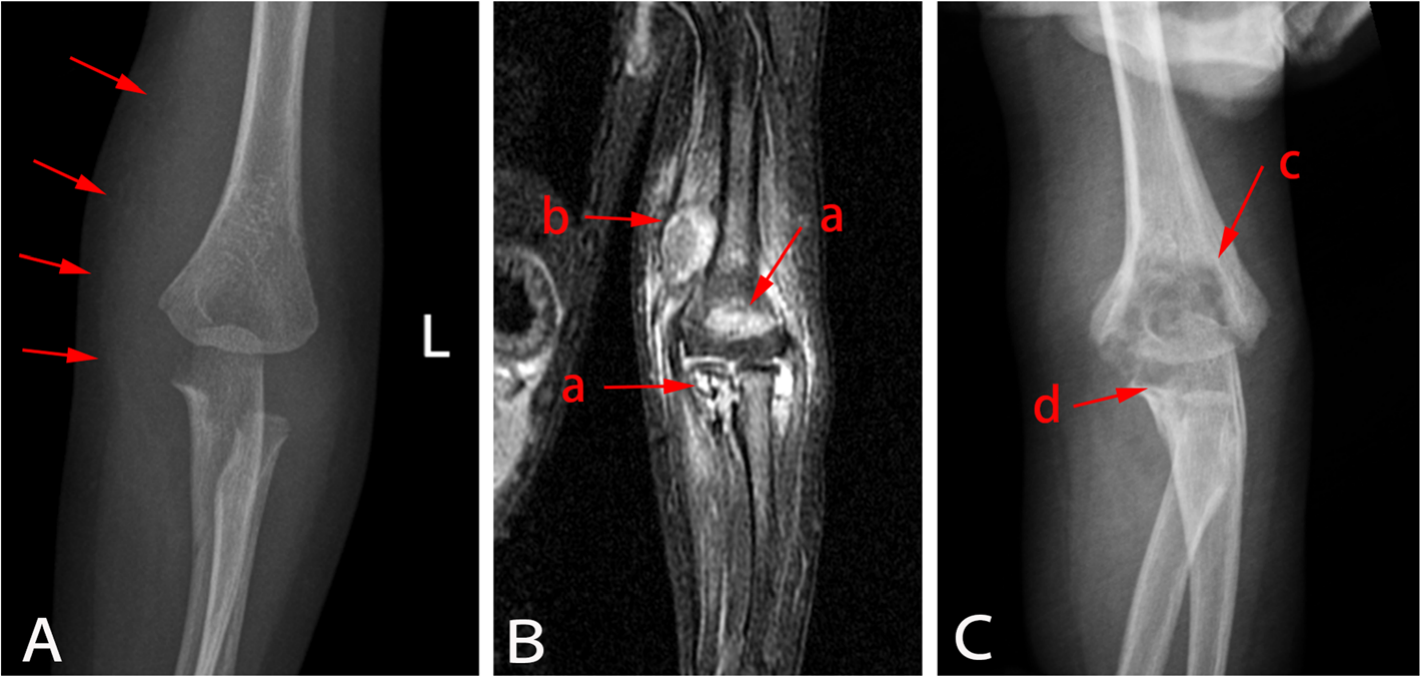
X-ray and MRI images showing changes around the left elbow. (A) Day 29 of admission: X-ray showing swelling of soft tissue around the left elbow (arrow). (B) Day 47 of admission: Coronal T2-weighted MRI showing patchy hyperintensity in the left humerus and proximal ulna (a) accompanied by soft tissue edema and abscess formation around the elbow joint (b).(C) Day 96 of admission: X-ray showing low-density shadows in the left humerus, suggesting bone destruction (c). Similar less severe changes were observed in the proximal left ulna (d). Soft tissue swelling was reduced
